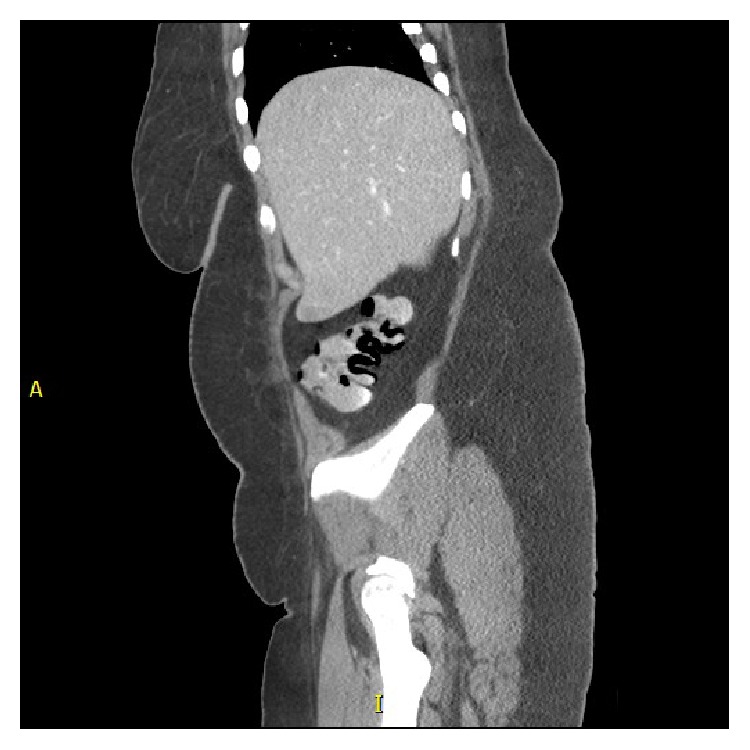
Showing Pneumatosis Intestinalis in right lower quadrant in a sagittal cut of CT imaging.
